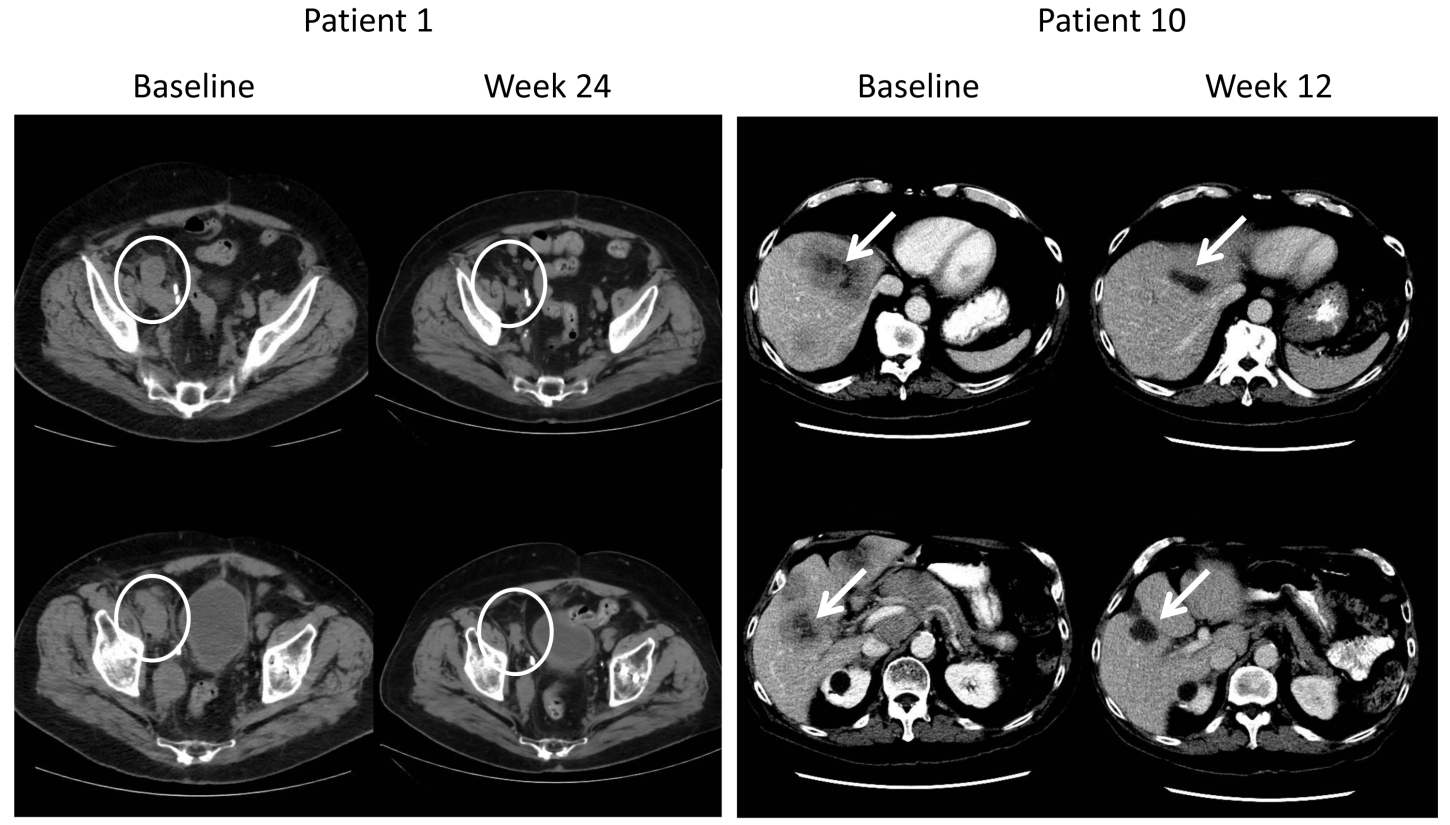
Radiographic Responses in Patients With Measurable Disease
